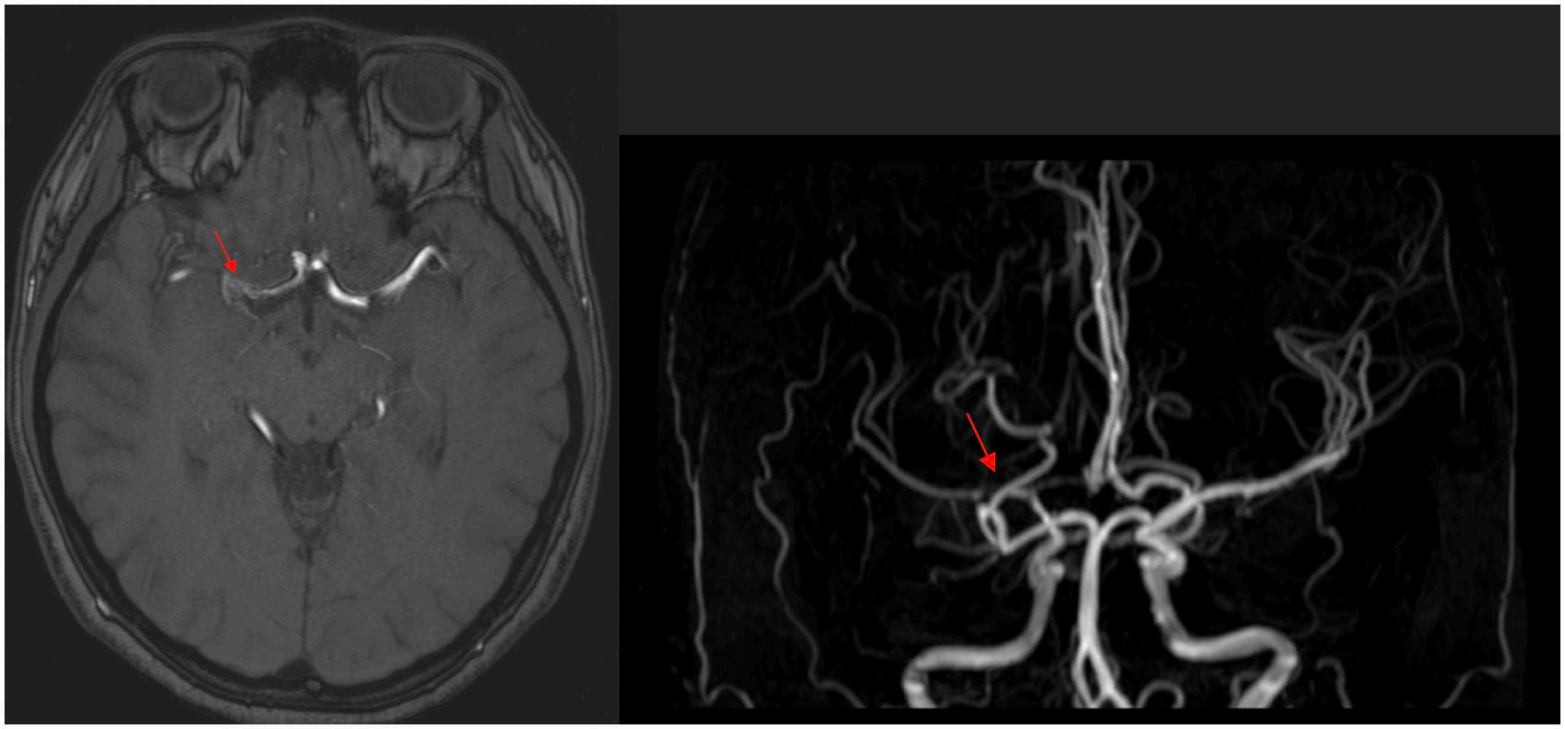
T1-weighted and 3D time-of-flight (TOF) show severe stenosis of M1, M2 segment of right middle cerebral artery (red arrow). No narrowing noted in contralateral side.
